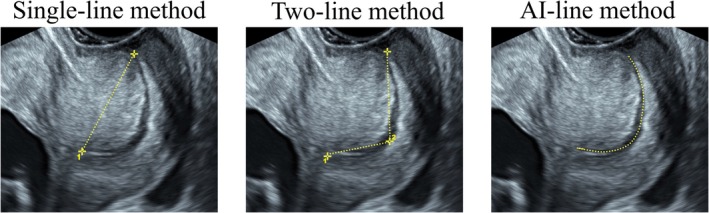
Transvaginal ultrasonographic CL measurements in the first trimester of pregnancy. (a) Single‐line method, as a straight line from the internal os to the external os. (b) Two‐line method, as the sum of two lines: One from the internal os to the greatest curvature, and the other from the end point of the first line to the external os. (c) AI‐line method, the length of the cervix by utilizing the scale between the internal and external cervical os (two ends of the glandular area around the endocervical canal) provided by tracing the endocervical canal to determine the actual length of the cervix. AI, artificial intelligence; CL, cervical length.
